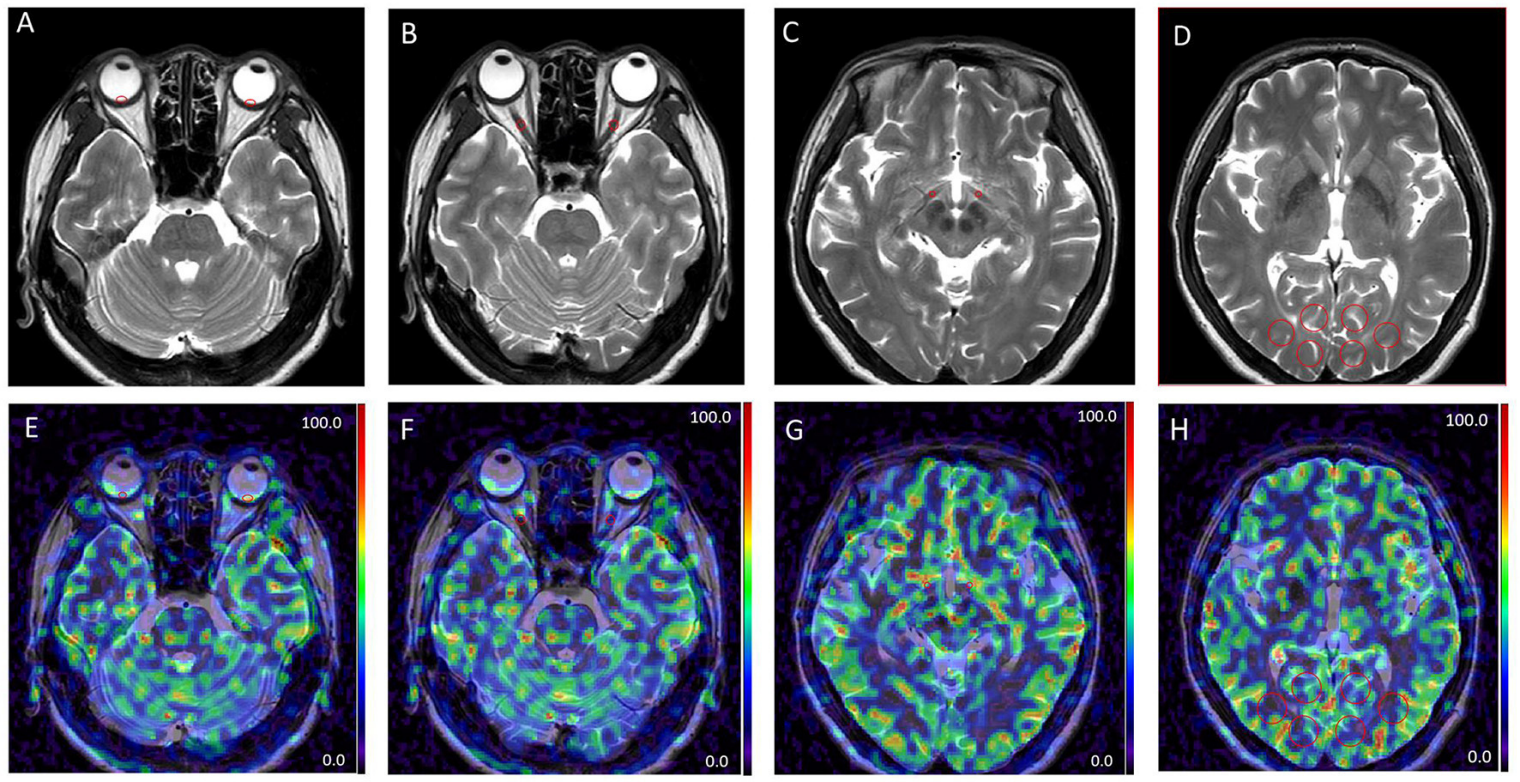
Examples of different patterns in MRI images. T2 weighted images (A–D). Arterial spin labeling (ASL) images at post-labeling delay (PLD) of 1.5 s (E–H). Regions of interest derived from the retinal-choroidal complex (A, E); the intraorbital segments of the optic nerve (B, F); the tractus opticus (C, G); the visual center (D, H); ROIs were all marked by red circles.
